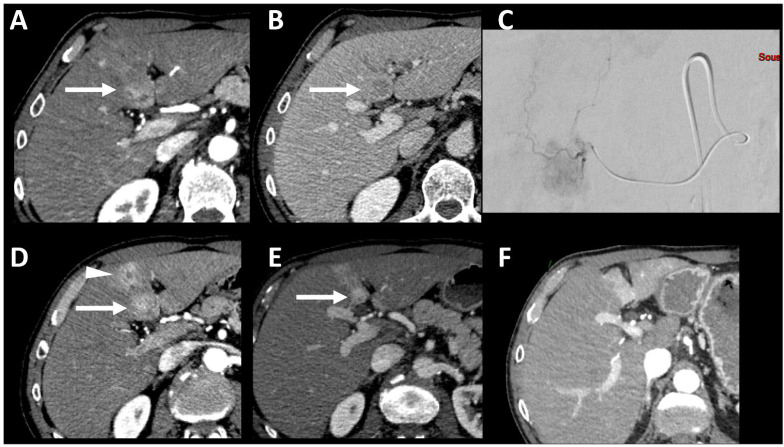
Imaging follow-up appearance after TARE for a hepatocellular carcinoma of the segment IV of the liver in a 61-year-old male. Pretreatment contrast-enhanced CT images (A,B) obtained during hepatic arterial phase (A) and portal venous phase (B), show a hepatocellular carcinoma in the segment IV (arrows). Digital subtraction angiography image (C) shows selective catheterization and injection of ^90^Yttrium microspheres in the segmental branch of the segment IV. 3-, 6- and 12-month follow-up CT images ((D–F), respectively) obtained during arterial phase show a slow progressive reduction in the lesion size and a persisting arterial phase hyperenhancement at 3 and 6 months ((D,E) arrows). Note the appearance of a peritumoral arterial enhancement 3 months after treatment ((D) arrowhead), which should not be misinterpreted with tumor progression, as well as the progressive atrophy of the segment IV, which is almost complete at 12 months (F).
